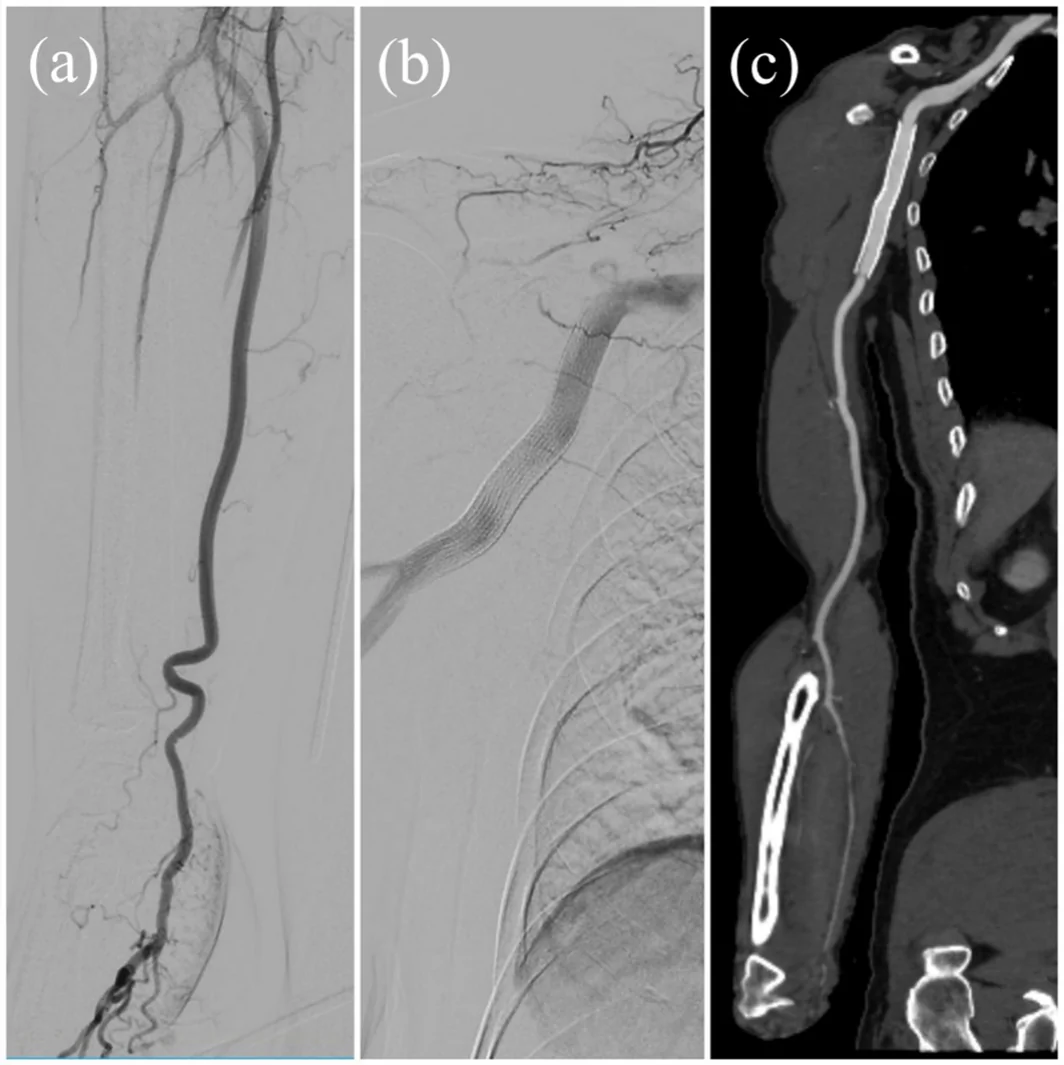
Angiography after thrombolysis, stent deployment and 2‐month follow‐up CTA. (a) Angiography after 3 days of catheter thrombolysis: Complete thrombus clearance with unobstructed distal perfusion; (b) Intraoperative angiography after covered stent graft implantation: A 10 × 80 mm Viabahn stent deployed to exclude axillary artery aneurysm, no endoleak observed; (c) Two‐month follow‐up CTA: Patent axillary stent without migration or endoleak, sustained patency of distal upper limb arteries.
